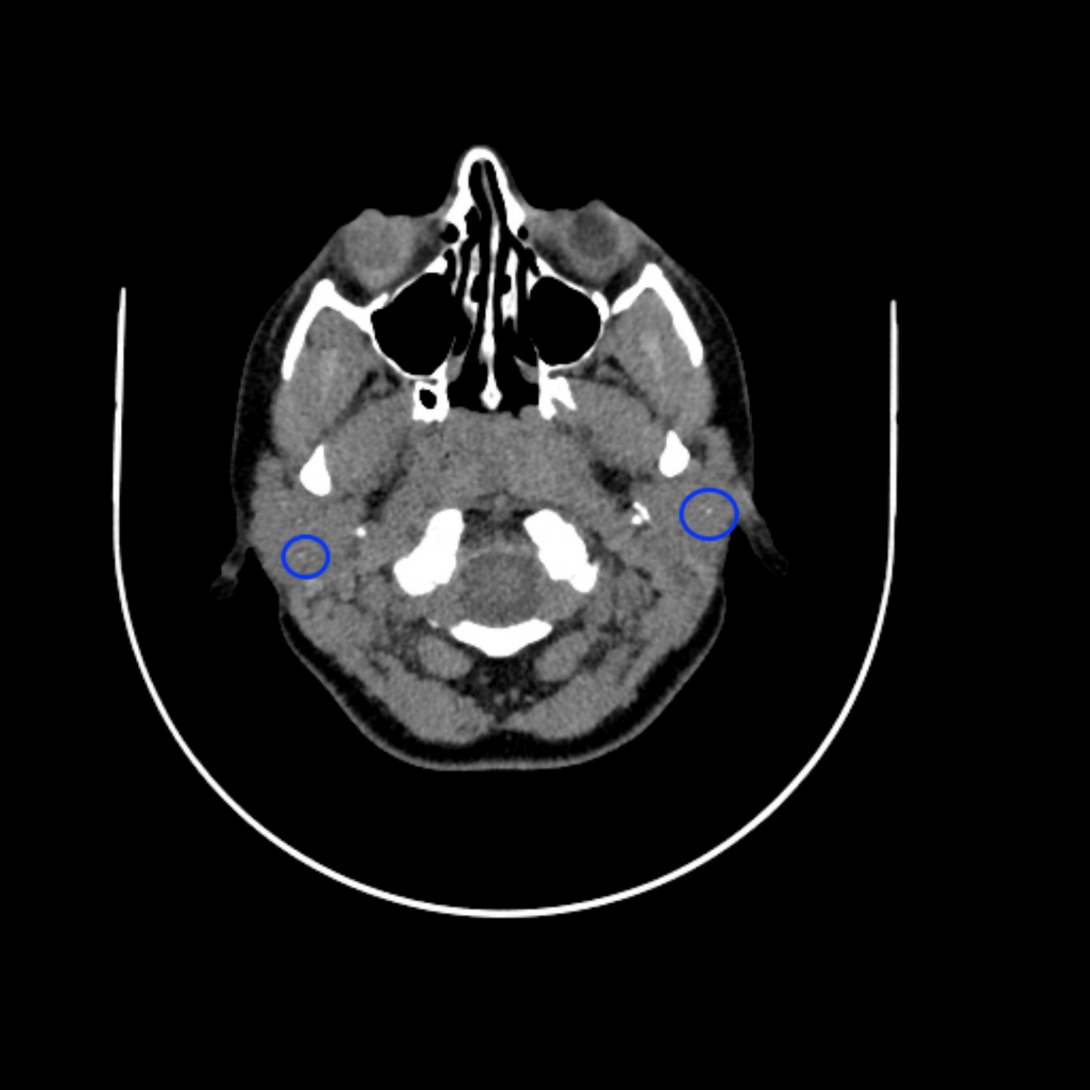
Precontrast CT shows tiny foci of calcifications within the parotid gland bilaterally. Foci of calcifications are encircled by blue rings. Precontrast CT shows tiny foci of calcifications within the parotid gland bilaterally. Foci of calcifications are encircled by blue rings.
